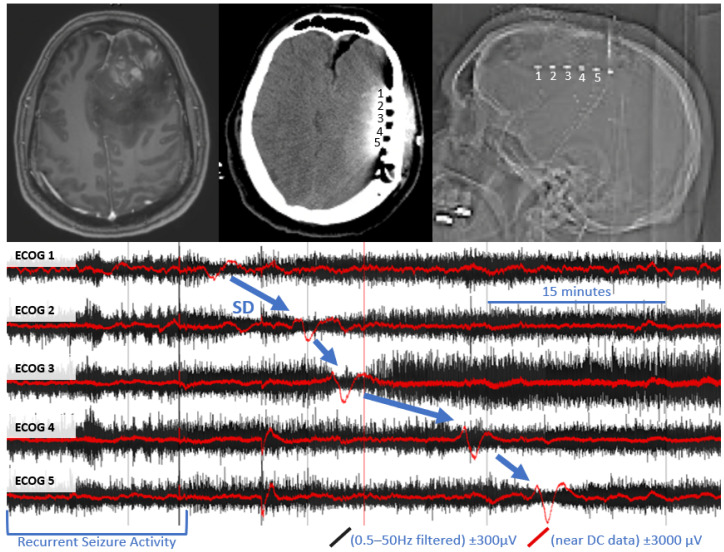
Example of spreading depolarization in a patient with high grade glioma. Upper left panel: pre-operative MRI with and without contrast, demonstrating contrast enhancing left frontal mass with surrounding vasogenic edema. Upper middle panel: post-operative CT, demonstrating the location of the subdural electrode (labeled 1–5) posterior to the tumor resection bed. Upper right panel: scout film from CT also demonstrating the location of the electrode. Bottom panel: referential traces from contacts 1–5 (1 is closest to tumor bed). The black traces represent time compressed, high frequency ECoG data, filtered a 0.5–50 Hz. Overlying red traces represent the same data near DC traces (>0.005 Hz for baseline leveling). A propagating wave of DC shift/slow potential change (in red) that is accompanied by transient suppression of high frequency signaling (in black) is demonstrated (blue arrows).
